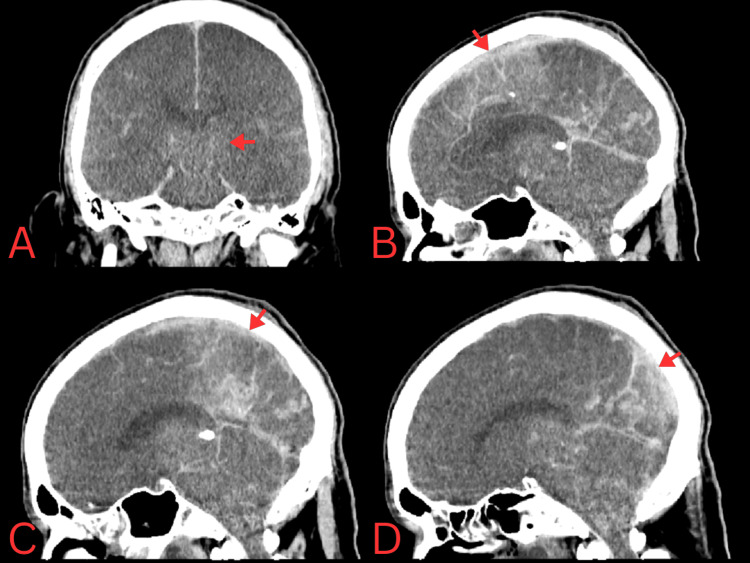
Third non-contrast CT brain demonstrating severe diffuse cerebral edema (A) Coronal view showing diffuse loss of gray-white matter differentiation and effacement of cerebrospinal fluid spaces, including the basal cisterns. (B-D) Sagittal views demonstrating diffuse cerebral swelling with sulcal effacement and compression of the ventricular structures. Hyperattenuation within the basal cisterns and cortical sulci was noted, raising concern for subarachnoid hemorrhage versus pseudo-subarachnoid hemorrhage in the setting of severe cerebral edema. Arrows indicate representative areas of cerebral swelling and hyperattenuation.
